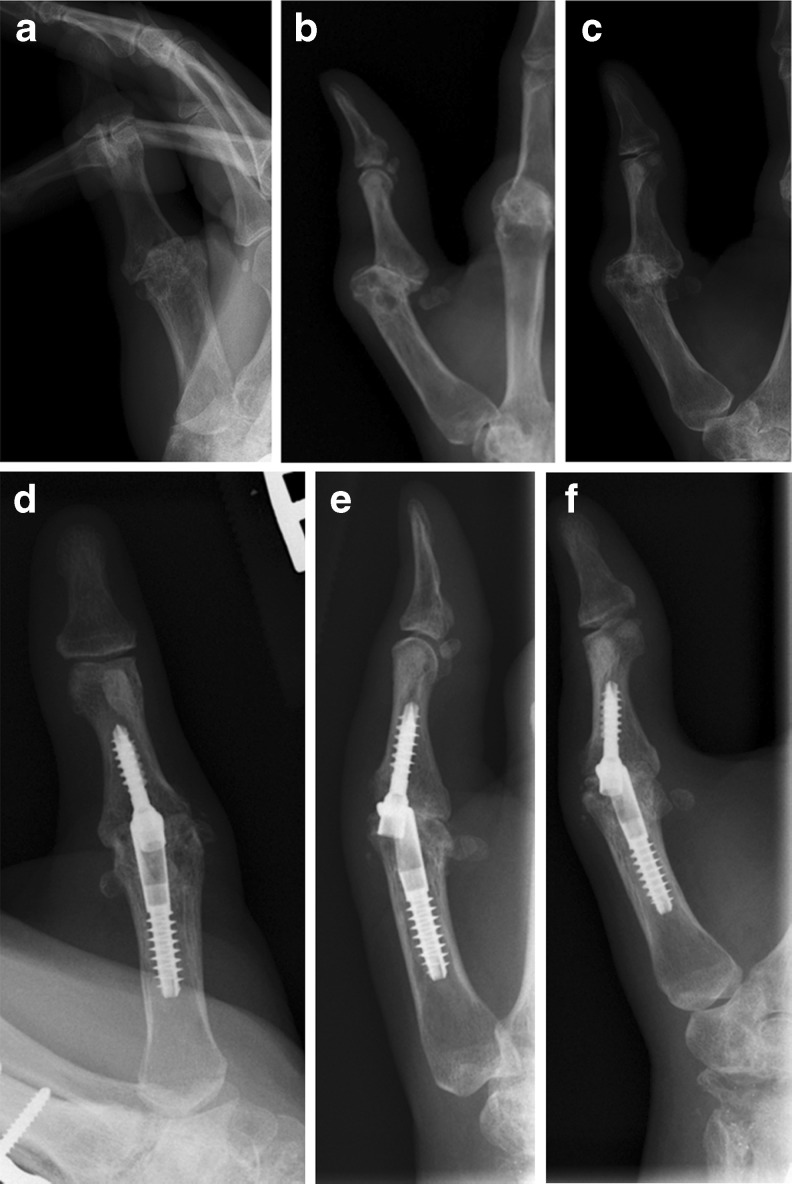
a Preoperative AP radiograph, right thumb of 40 yo female with rheumatoid arthritis and metacarpophalangeal joint instability and arthritis (Pt. 9). b Preoperative lateral radiograph, right thumb of Pt. 9. c Preoperative oblique radiograph, right thumb of Pt. 9. d Postoperative (5 weeks) AP radiograph, right thumb of Pt. 9 showing intramedullary compression device and fusion of metacarpophalangeal joint. e Postoperative (5 weeks) lateral radiograph, right thumb of Pt. 9 showing intramedullary compression device and fusion of metacarpophalangeal joint. f Postoperative (5 weeks) oblique radiograph, right thumb of Pt. 9 showing intramedullary compression device and fusion of metacarpophalangeal joint
